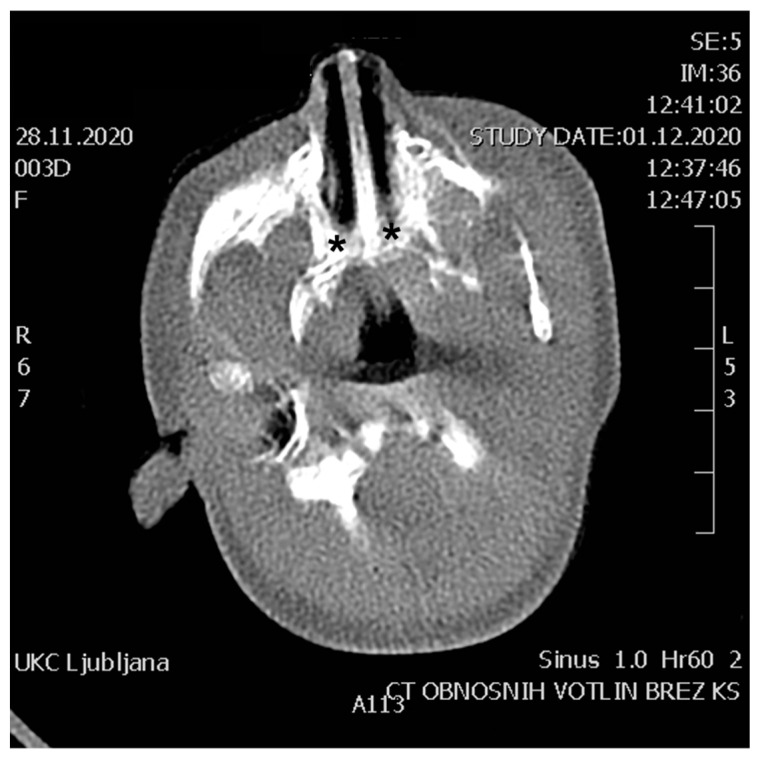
CT of the nose and paranasal sinuses in the axial plane. Bilateral bony choanal atresia is seen in a three-day-old neonate. * indicates atretic plate.
